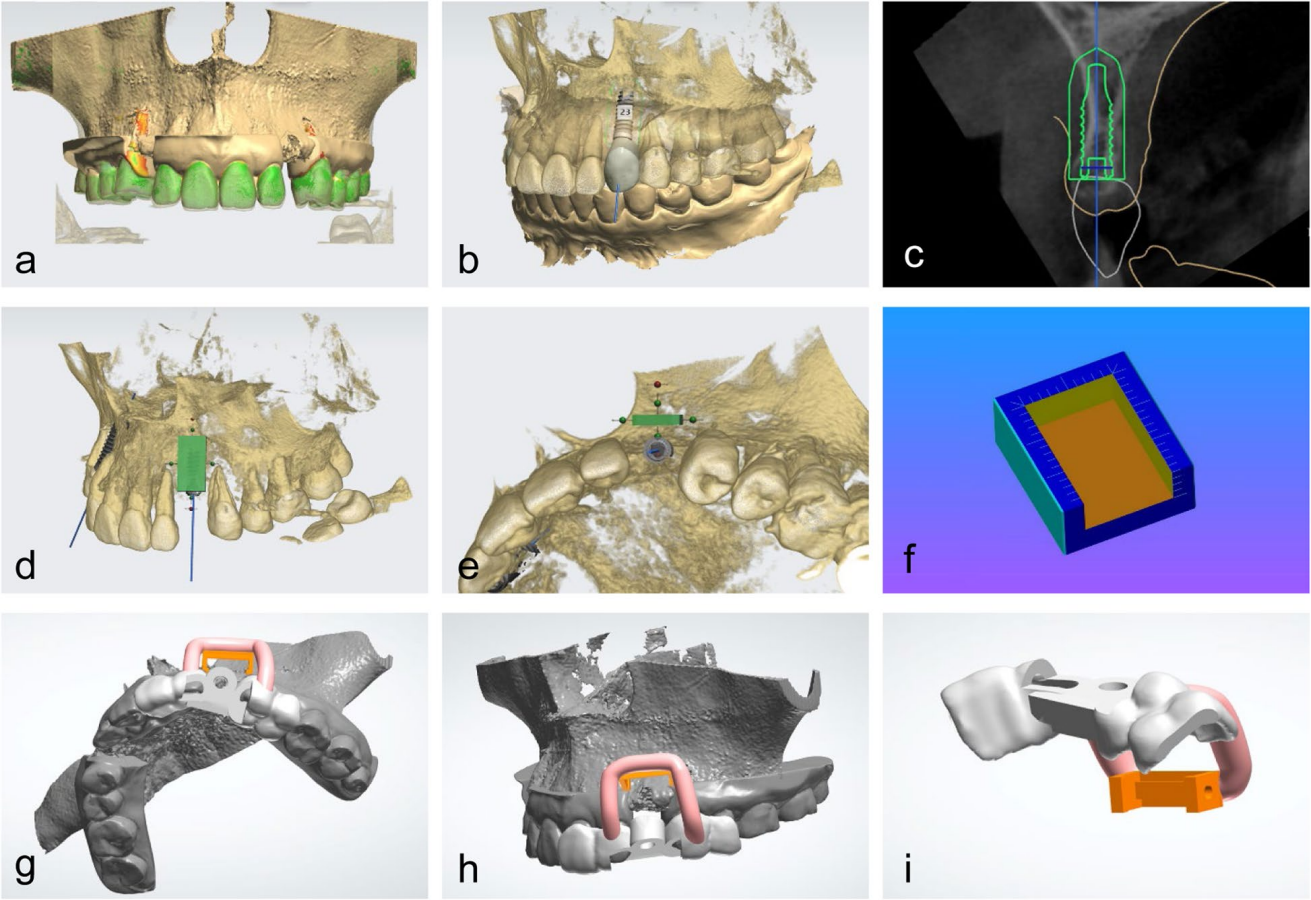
Design of definitive prosthesis, implant, bone block and template. a Dentition scan model superimposed onto 3-dimensional reconstructed maxilla; b planned definitive prosthesis and implant; c planned prosthesis (white) and implant position (green) on CBCT; d-e planned position of the bone block graft (green); f designed bone trim template; g-i designed bone graft template, including tooth-supported base template (white), clamp (orange) and rod-like attachment (pink)
